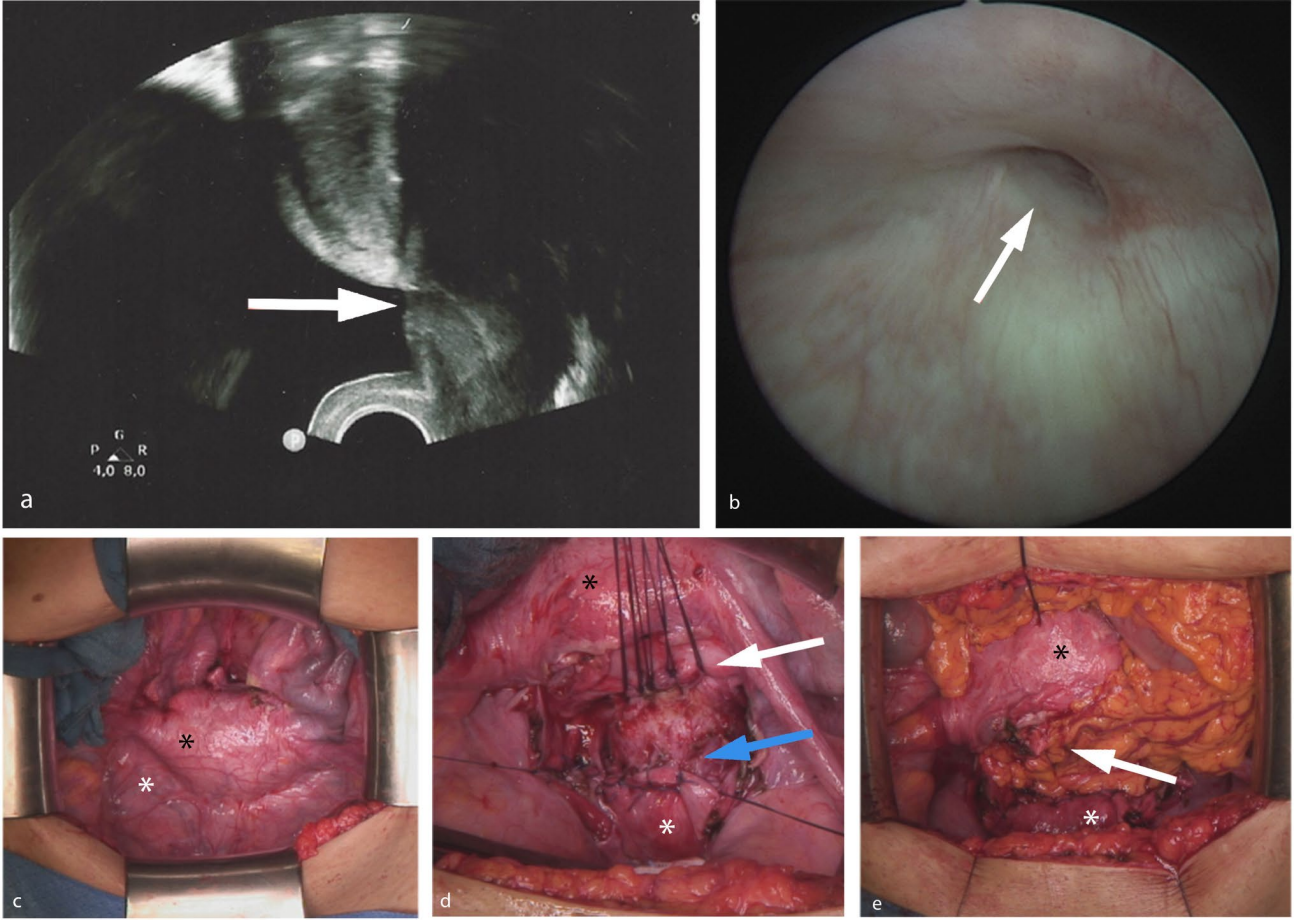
a Pelvic floor sonography: fistulous tract between the posterior bladder wall and the isthmus uteri (white arrow); b cystoscopy: fistula opening (white arrow); c laparotomy: uterus (black asterisk), bladder (white asterisk), d the fistula openings were closed (uterus suture-line white arrow, bladder suture-line blue arrow), e omental flap interposition between the uterus and the bladder (white arrow)
